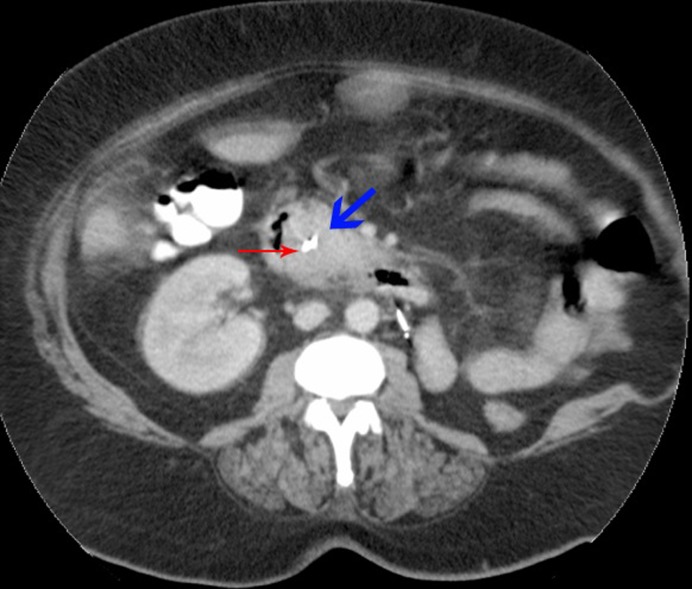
Cross-section of CT abdomen: blue arrow - tumor deposit; red arrow - biliary stent, D2 of duodenum to the right.
